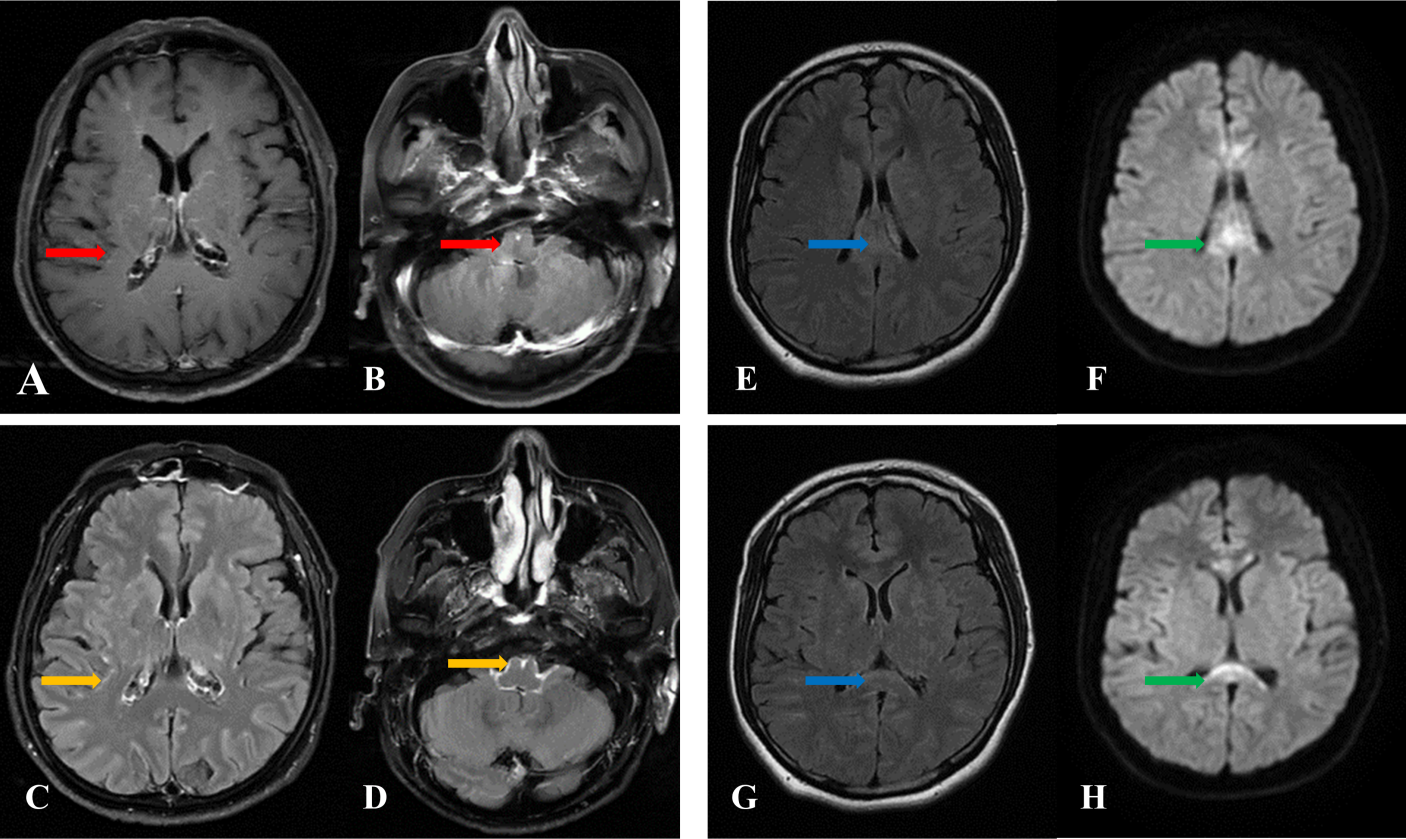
(A, B) Axial T1 gadolinium contrast enhanced MRI of the brain, patient #8 (C, D) Axial T2 weighted fluid attention (T2-FLAIR) post contrast, demonstrating linear intraparenchymal and leptomeningeal enhancement. (E, G) Axial T2-FLAIR, and (F, H) diffusion weighted imaging (DWI), with patchy T2-FLAIR and DWI hyperintensity in the corpus callosum, patient #1.
